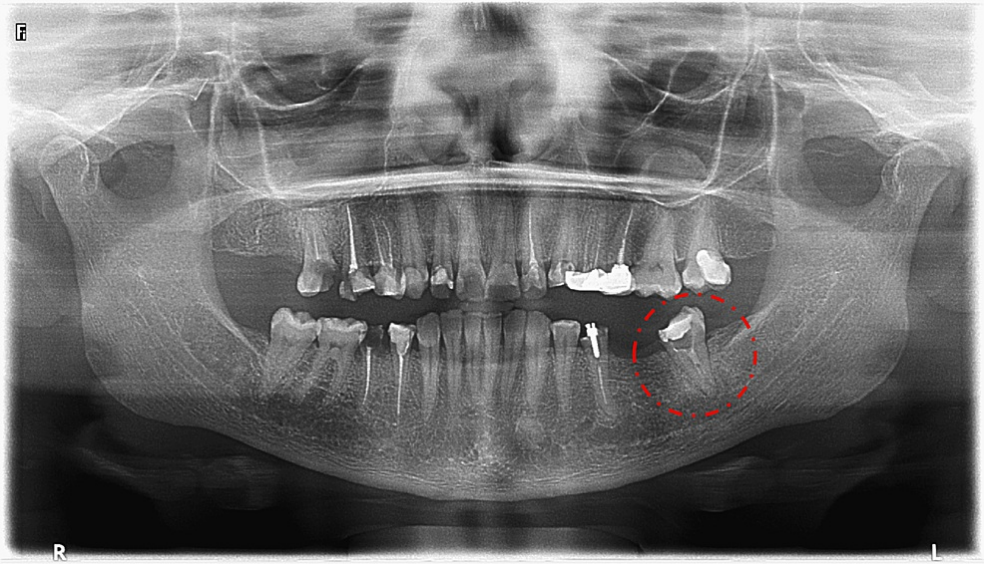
Orthopantomogram (OPG) radiograph
